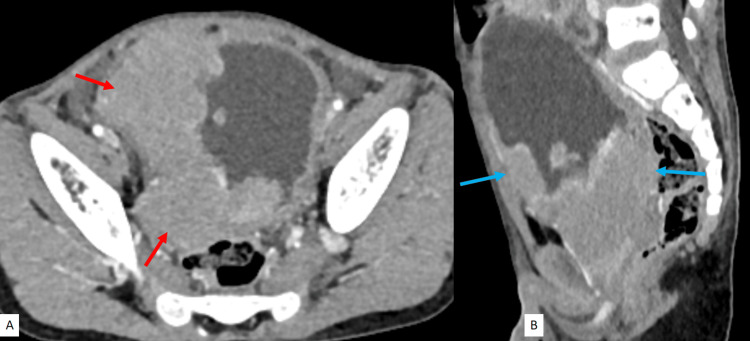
CECT scan of the abdomen: (A) Axial section showing a homogeneously enhancing mass arising from the right lateral wall and trigone of the urinary bladder (red arrows). (B) Sagittal section demonstrating anterior and posterior perivesical extensions of the mass (blue arrows). CECT: contrast-enhanced computed tomography
